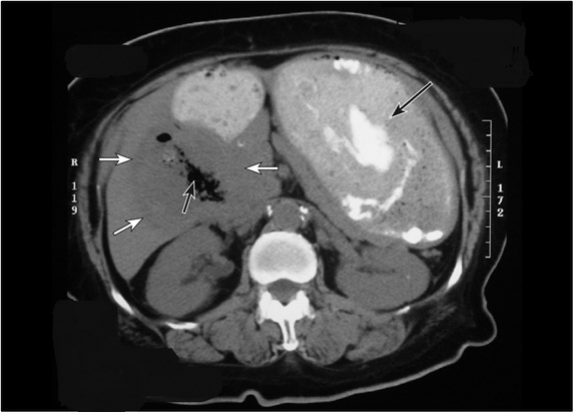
Computed tomography (CT) scan of the abdomen showing distended stomach compatible with gastric outlet obstruction (long black arrow), gallbladder fossa abscess (area of different density inside the liver) (white arrows), and free air inside the fossa (short black arrow).
